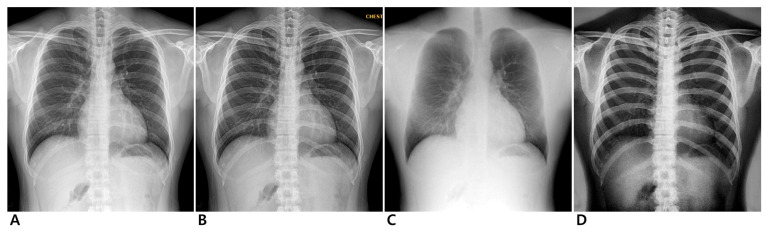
Chest radiographs of 24-year-old male participant. Conventional (A), enhanced standard (B), soft-tissue-selective (C), and bone-selective (D) images.
